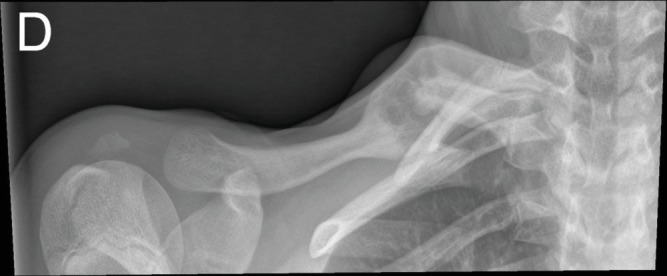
X-ray imaging showing partial callus formation, hypertrophic nonunion, and deformation at the boundary of the midshaft and proximal right clavicle.
